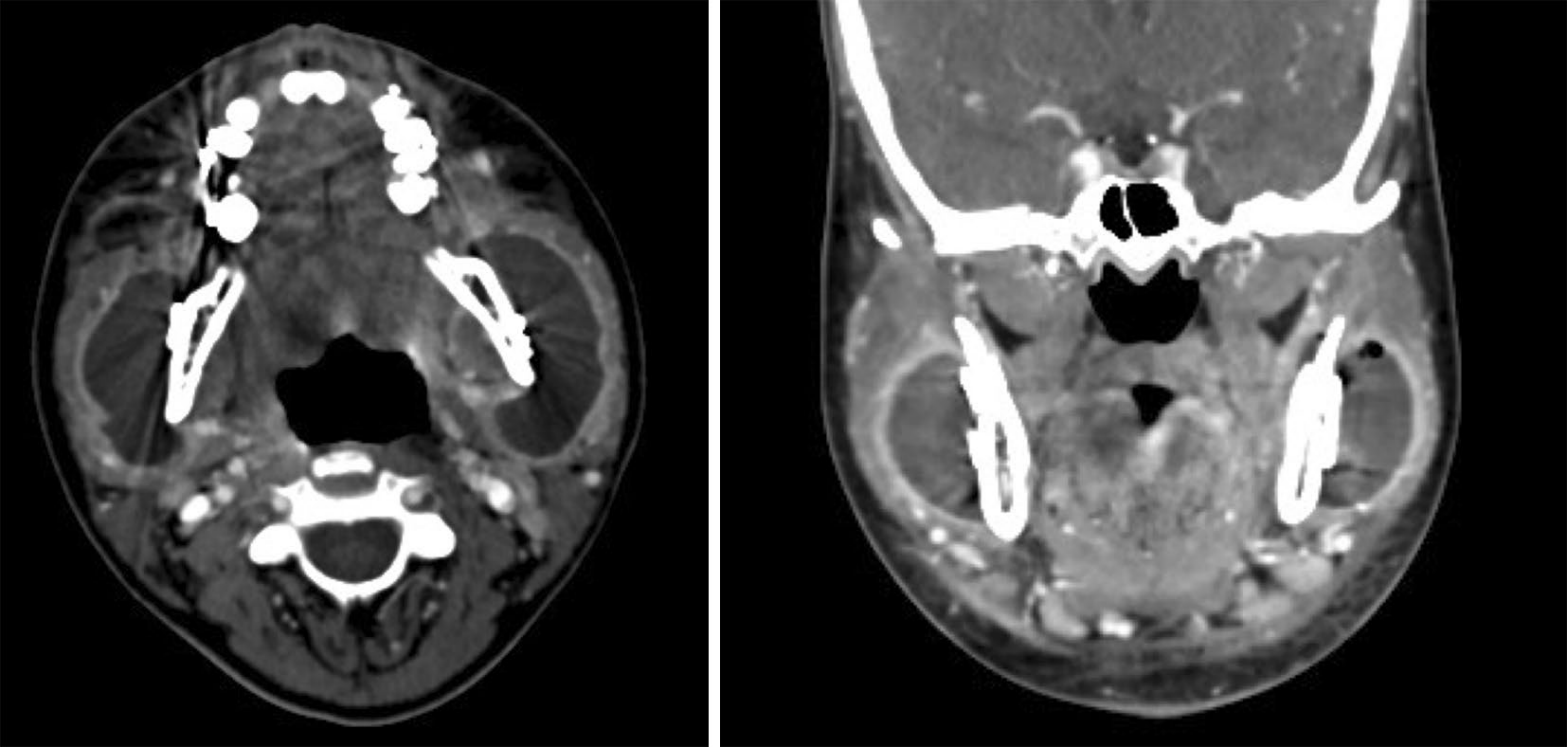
Extensive cellulitis with purulent collection after bilateral sagittal split osteotomy (CT scan, axial and coronal views).
